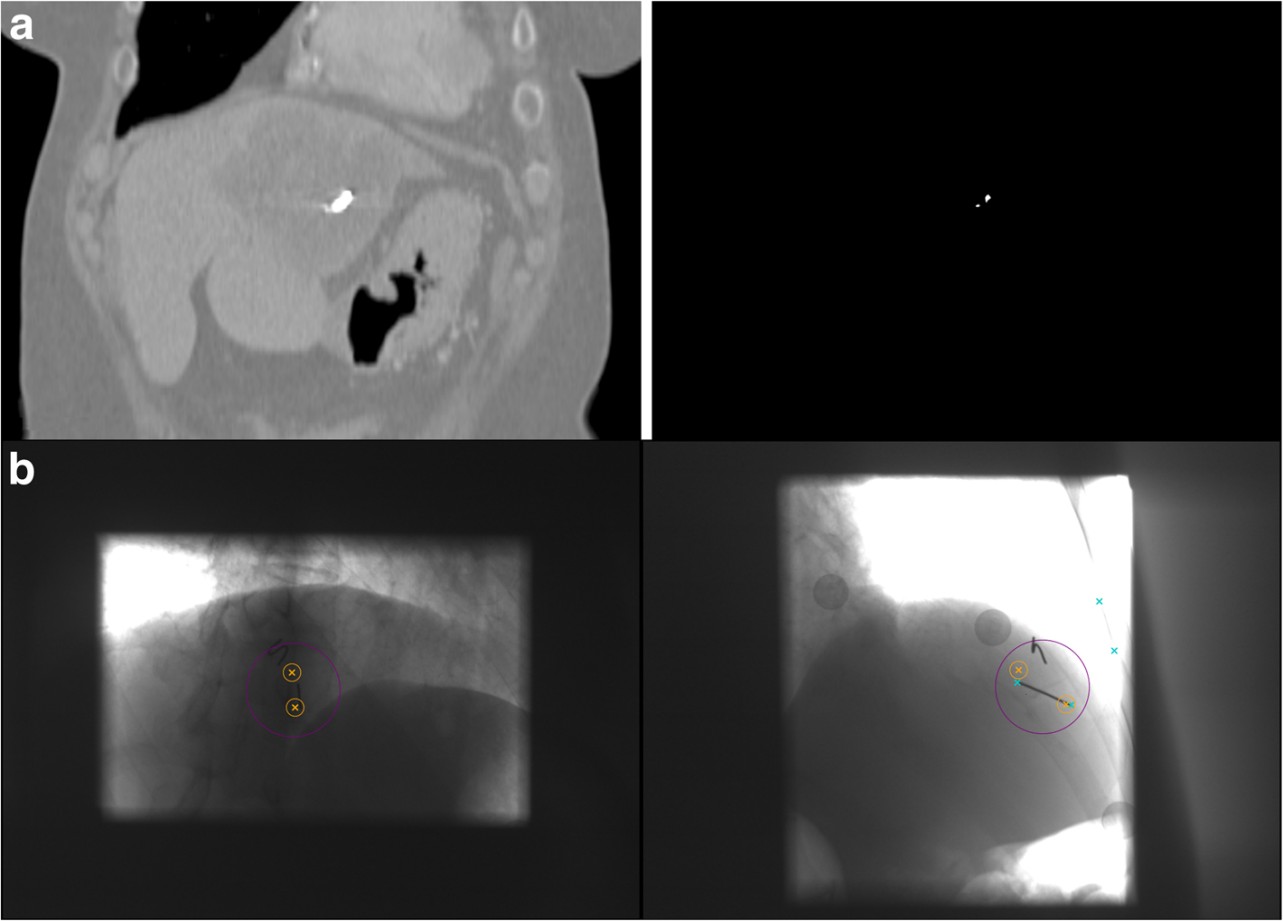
Part a shows an example of the extreme windowing used for the marker definition. The endpoints can be seen much clearer in the strong windowing. Part b shows X-ray images (window 4500, level 4500) of the ExacTrac system with the predicted marker position in orange and the possible detected positions in blue. The orange circle surrounding the predicted position has a radius of 3 mm. In this image the FM detection was only successful for *imager2* (right) with a surrounding intensity (purple circle) of over 5000. The dark borders on the edges come from the partly closed jaws of the imagers. The second marker visible within the image was not defined within the ExacTrac since it is strongly bent. Thus there is no predicted position for this marker
